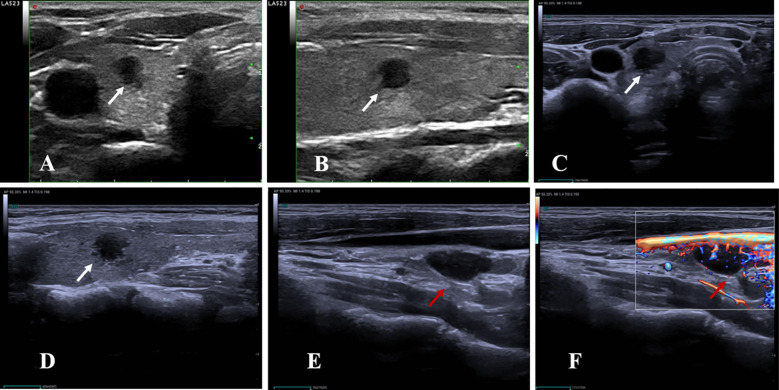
Lymph node metastasis and meeting the ≥3 mm maximum diameter progression criterion during active surveillance of a highly suspicious thyroid nodule. A 35-year-old woman was initially diagnosed with a ACR TI-RADS 5 thyroid nodule. Baseline transverse (A) and longitudinal (B) ultrasound images show a suspicious nodule measuring 3.9 × 3.9 × 4.3 mm. At the 23-month follow-up, transverse (C) and longitudinal (D) images demonstrate notable growth of the original nodule to 7.0 × 5.8 × 7.1 mm, meeting the ≥3 mm maximum diameter progression threshold. A suspicious lymph node was simultaneously identified (E), with minimal intranodal vascularity seen on color Doppler imaging (F). Fine-needle aspiration confirmed the diagnosis of lymph node metastasis. White arrows indicate the original thyroid nodule; red arrows indicate the metastatic lymph node.
